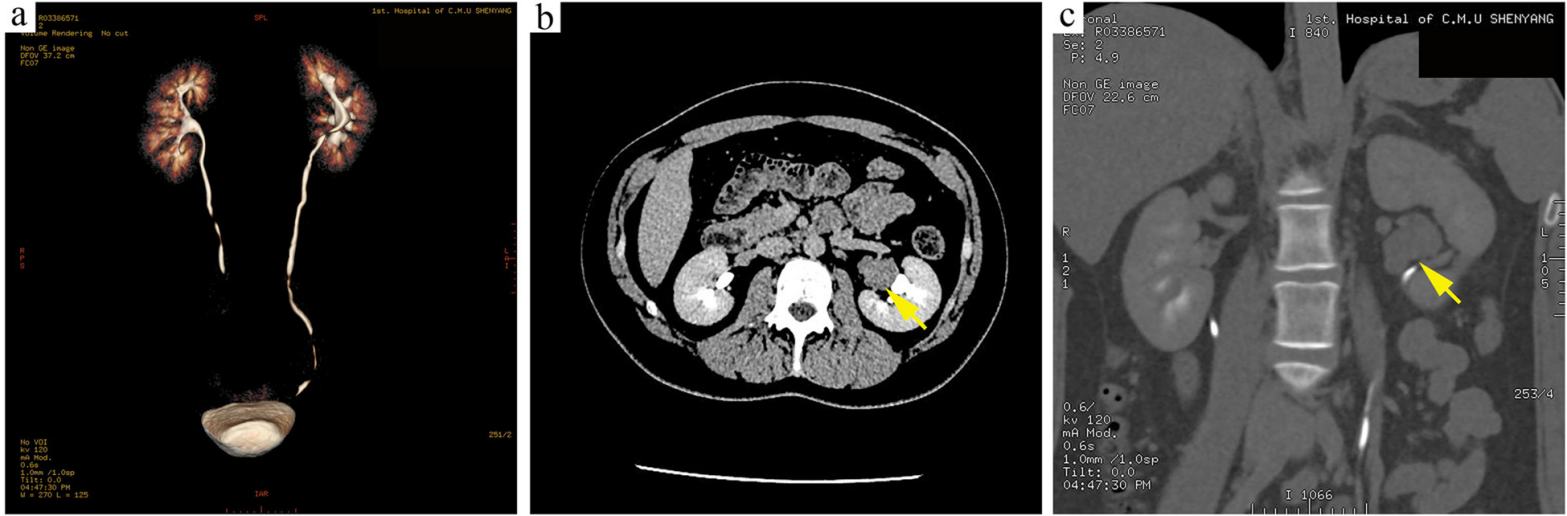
Imaging examination results of the patient. a Computed tomography (CT) urography and three dimensional reconstruction show the ureters bilaterally, without any obvious expansion. Enhanced CT and coronal reconstruction showing b a soft-tissue mass with a maximum diameter of 2.7 cm in the left renal hilum, near the renal pelvis and c an atrophic bilateral adrenals in sagittal view
